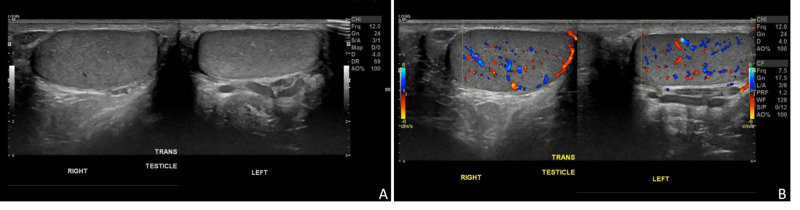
Ultrasound Evaluation Grayscale (A) and color Doppler (B) ultrasound images of the bilateral testes show no focal abnormality. This imaging examination is necessary to exclude a testicular primary in any suspicious or biopsy-proven case of extra-testicular germ cell tumors.
